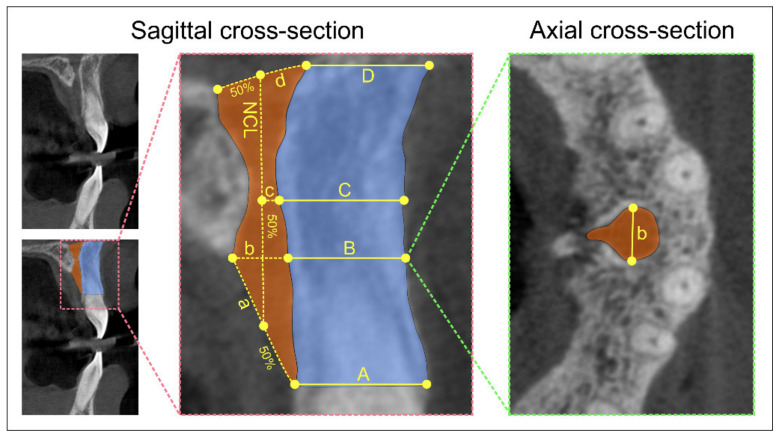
CBCT image and landmarks. Sagittal cross-section—sagittal CBCT slice (left, upper); slice with marked field of interest (left, bellow); selected landmarks for analyses (right)—(a) A-P diameter of the incisive foramen, (b) level for M-L measurement of the incisive foramen, (c) mid-length of the NPC, (d) A-P diameter of the nasal foramen, NCL (NPC length), (A) horizontal dimension of the anterior maxilla from the buccal border of incisive foramen to the cortical layer, (B) horizontal dimension of the anterior maxilla from the buccal wall of the nasopalatine canal to the cortical layer using a horizontal line from the palatal border of the incisive foramen, (C) horizontal dimension of the anterior maxilla from the buccal border at the midpoint level of NCL to the cortical layer, (D) horizontal dimension of the anterior maxilla from the buccal border of nasal foramen to the cortical layer; axial cross-section—(b) M-L diameter of the incisive foramen.
